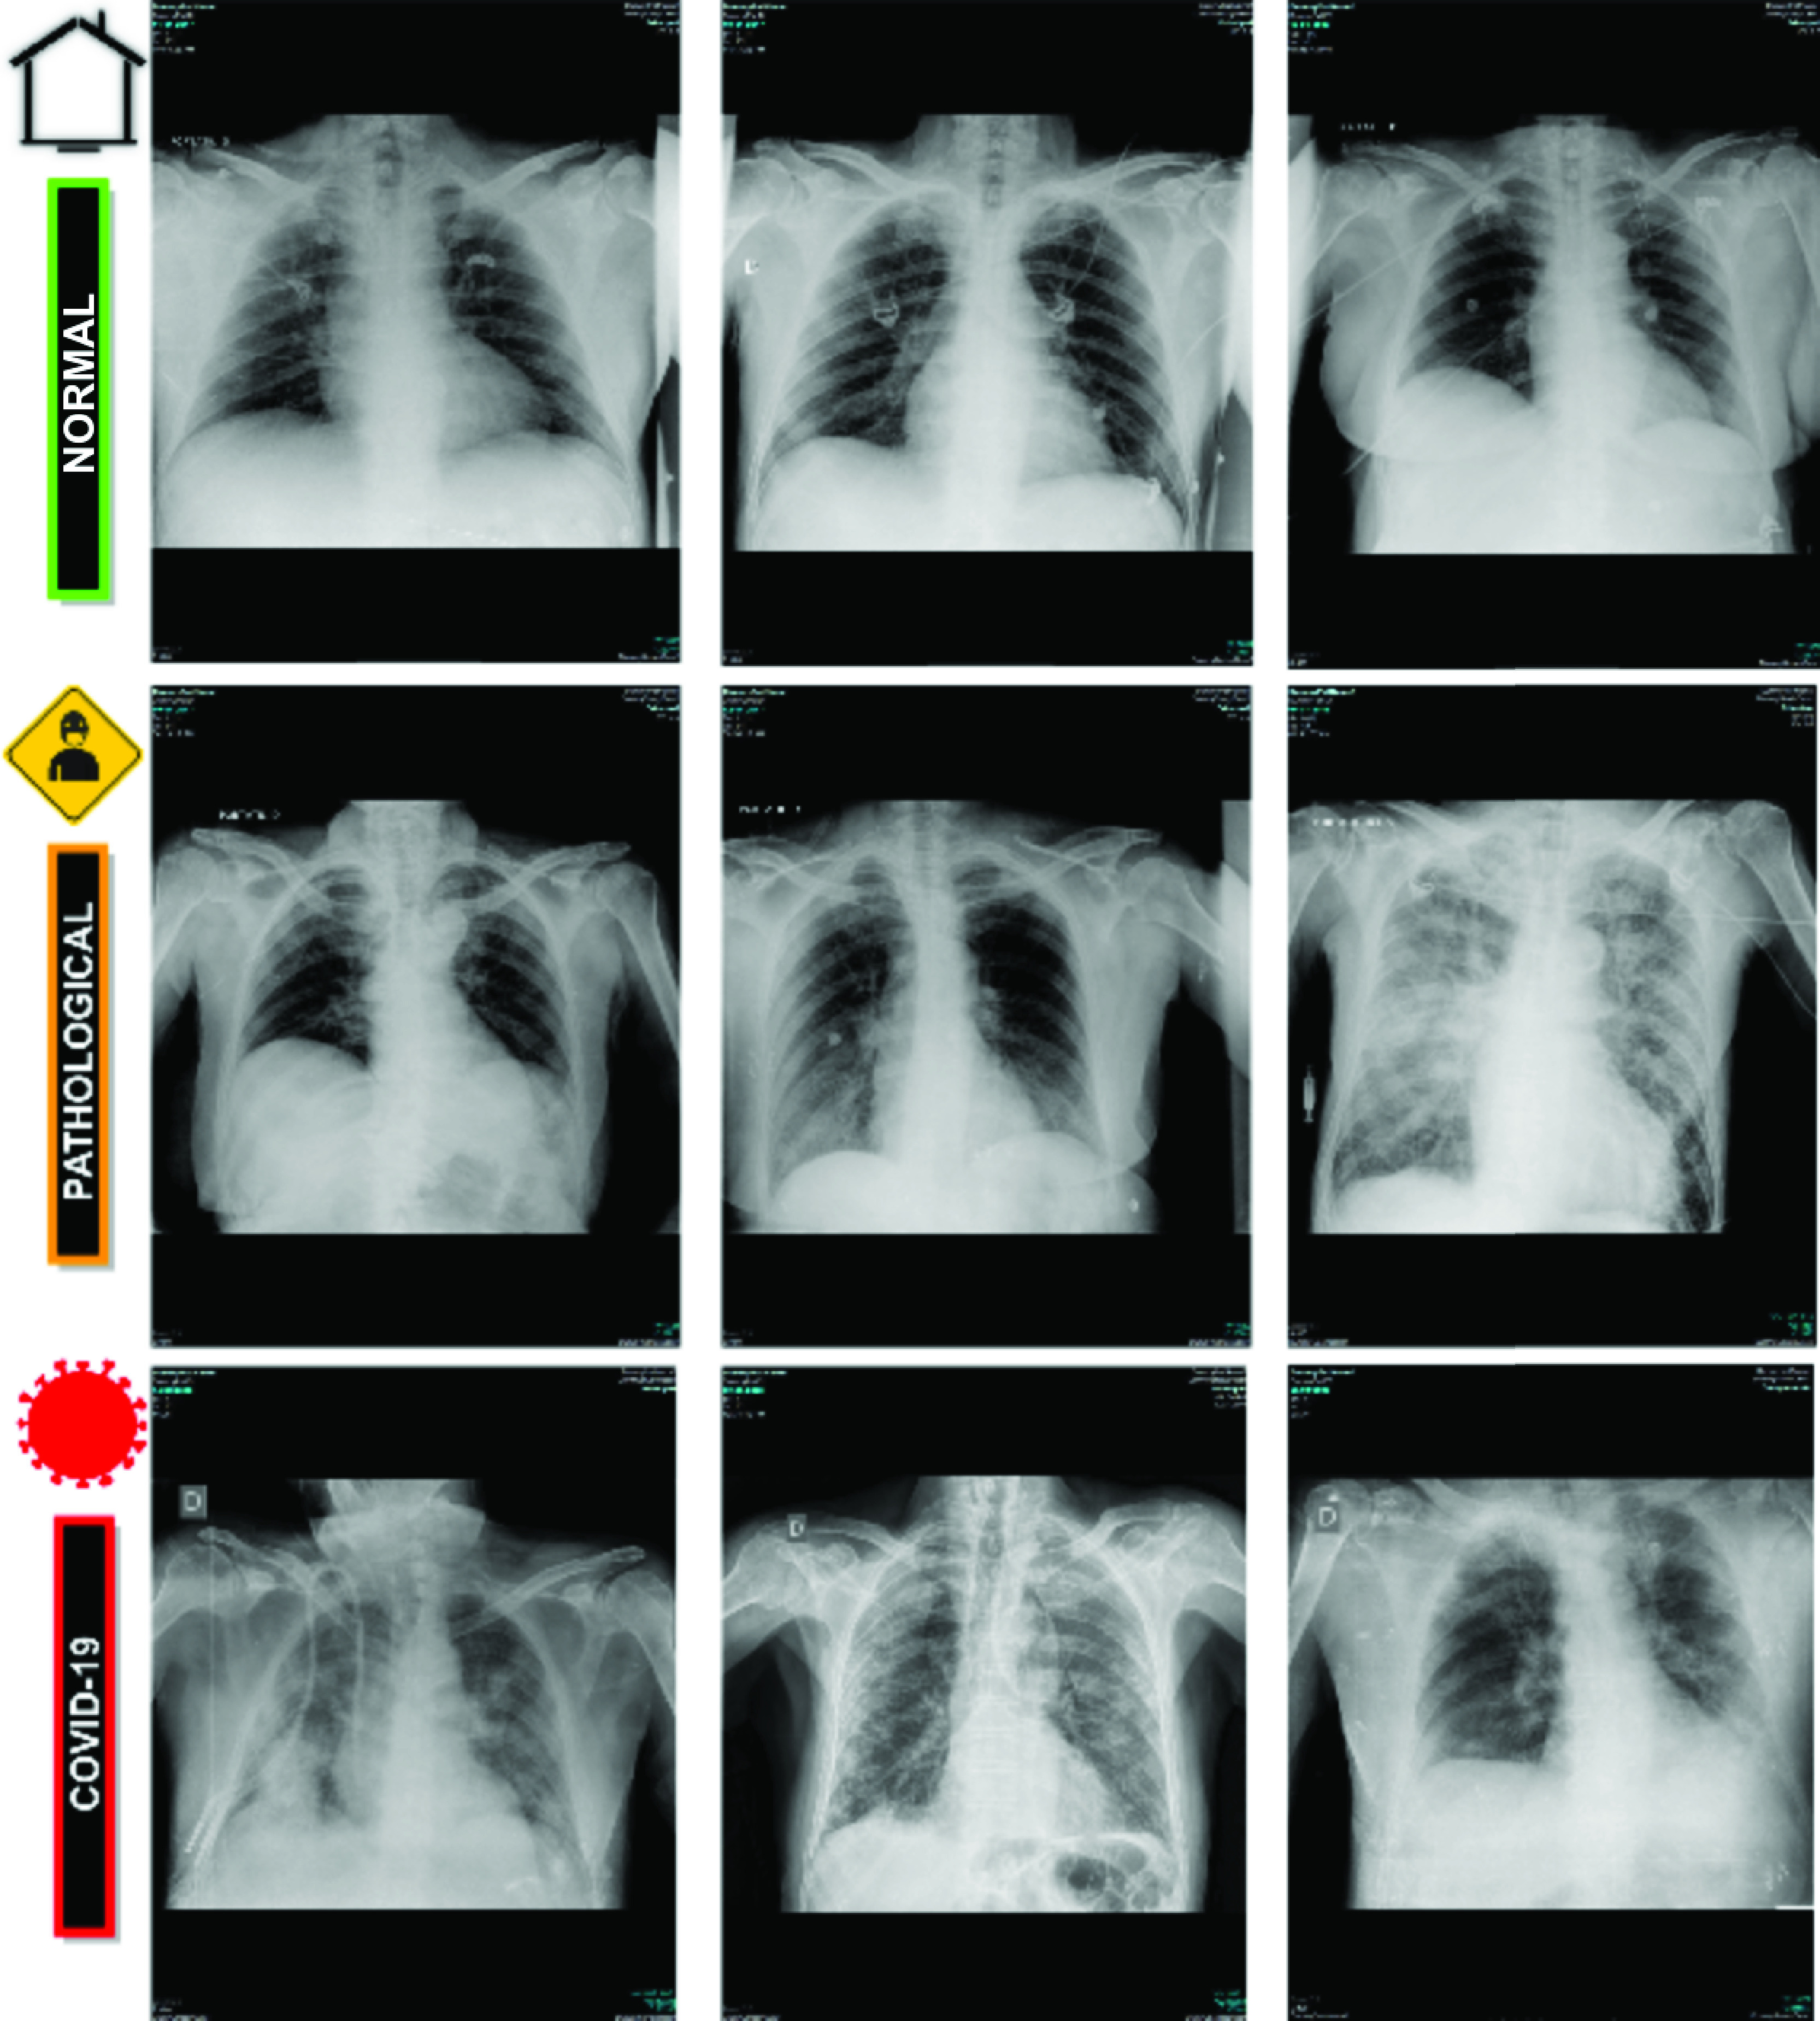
Representative examples of chest X-ray radiographs. }{}$1^{st}$ row, chest X-ray radiographs from normal patients. }{}$2^{nd}$ row, chest X-ray radiographs from pathological patients without COVID-19 but diagnosed with other pleural or pulmonary diseases. }{}$3^{rd}$ row, chest X-ray radiographs from patients with COVID-19.
